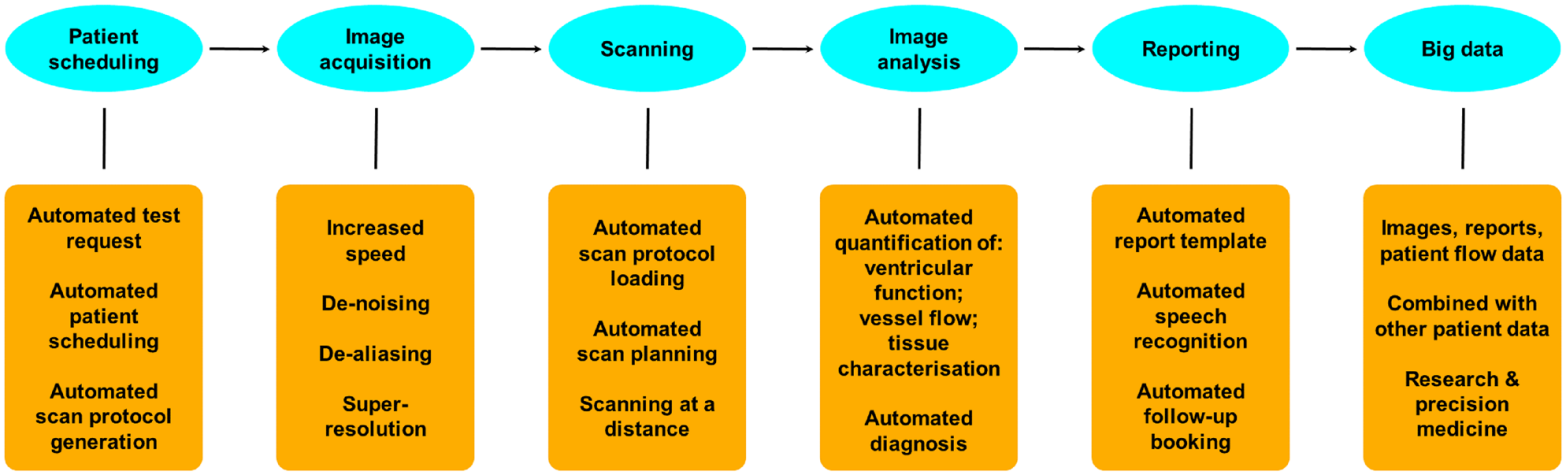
Aspects of the radiologic patient pathway for paediatric cardiovascular MRI where artificial intelligence can play a role
